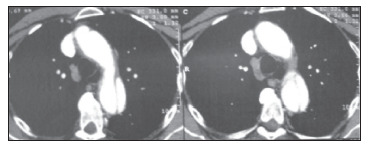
Chest computed tomography scan showing a false lumen in aorta (aortic dissection).
